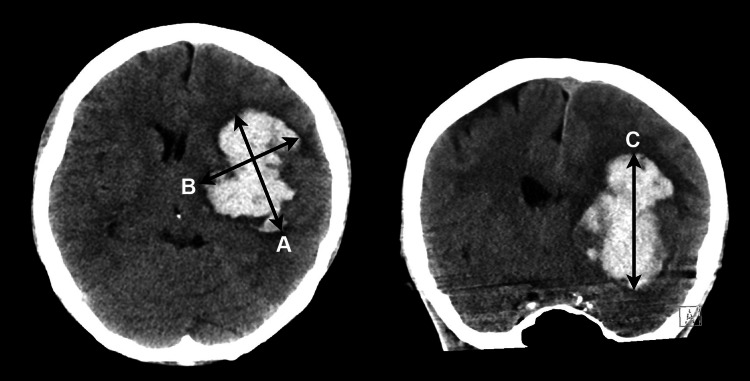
Representative computed tomography brain study image from the cohort demonstrating the ABC/2 measurement technique for hematoma volume estimation using the ellipsoid method with perpendicular diameters A, B, and C. A is the largest diameter of the hematoma on the index slice; B is the largest perpendicular diameter on the same slice; C is the sum of slice thickness contributions, assigning 1 for slices with hemorrhage ≥75% of the index‑slice area, 0.5 for 25-75%, and 0 for ≤25%. For 5 mm slices, the summed C is divided by 2. ICH volume (in cm³) is calculated as (A × B × C)/2, approximating the volume of an ellipsoid. ICH, intracerebral hemorrhage.
